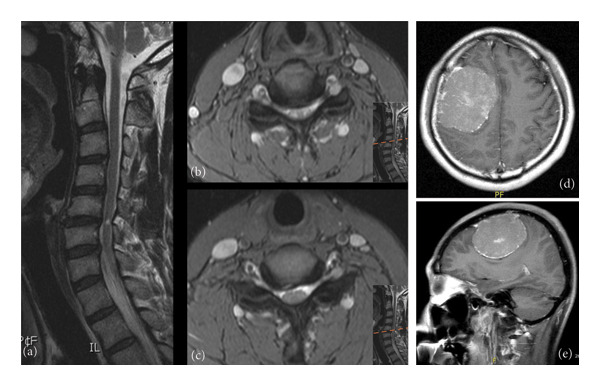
Case 2: Images of cervical magnetic resonance imaging. (a) Sagittal view showing disc bulging with canal stenosis at the C4–7 level. (b) Axial views of C4/5. (c) Axial view of C5/6. (d) Sagittal view of brain MRI. (e) Axial view of brain MRI revealed a well‐defined extra‐axial brain tumor measuring 7.5 cm in the right high frontal convexity area with a significant mass effect.
